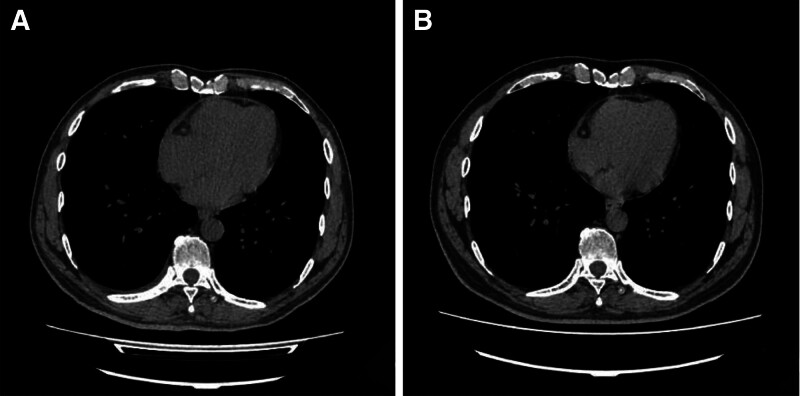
Follow-up chest computed tomography (CT) after treatment. (A) Axial mediastinal window CT image at 3 months after treatment shows a marked reduction in pericardial thickening compared to baseline. (B) Axial mediastinal window CT image at 9 months shows near-complete resolution of pericardial thickening, with no signs of calcification or effusion.
